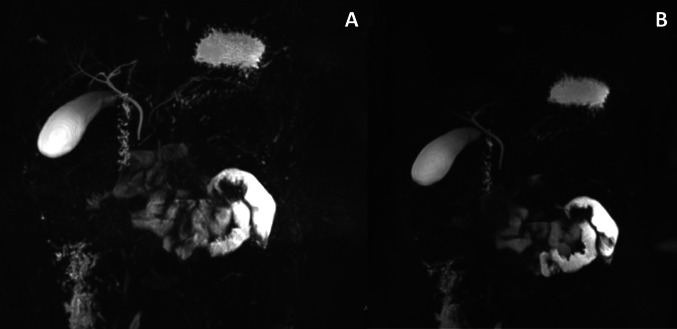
T2-weighted 3D SPACE MRCP exam of a 9 year old boy. “A” represents T2 3D SPACE MRCP acquisition using the respiratory triggering technique. “B” represents T2 3D SPACE MRCP image acquisition using the Breath-Hold technique
